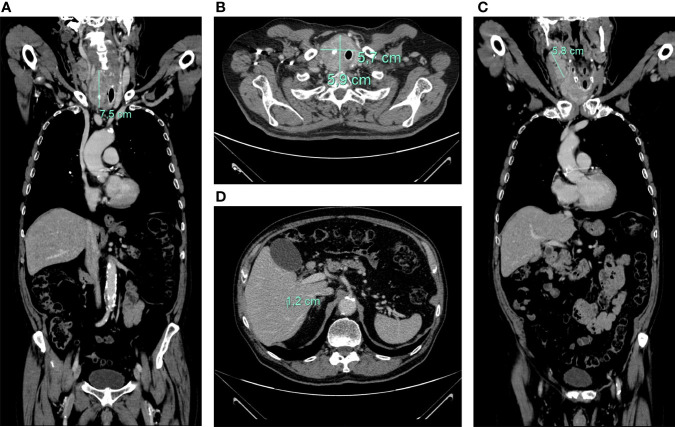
axial (A) and coronal (B) CT-scan showing large inhomogeneous tumor with calcifications of the right thyroid lobe with left tracheal displacement, infiltrating the trachea and esophagus; confluent neck lymph node metastases (maximum diameter of 58 mm) (C); hepatic lesion of 12 mm at V segment (D).
